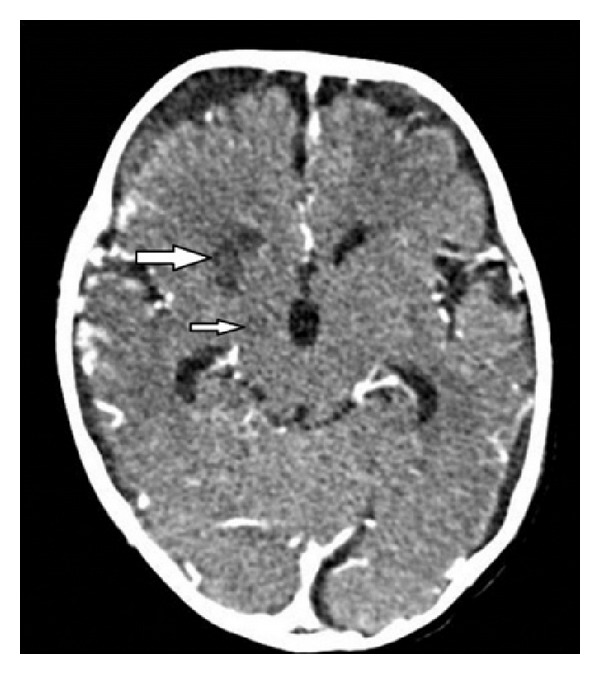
Enhanced brain computed tomography scan nine days after admission. Axial image through the basal ganglia shows hypodensities involving the right head of the caudate and anterior aspect of the putamen (thick white arrow) as well as the right hypothalamus (thin white arrow) representing subacute infarcts. In addition, there is development of bilateral hemispheric hygromas.
